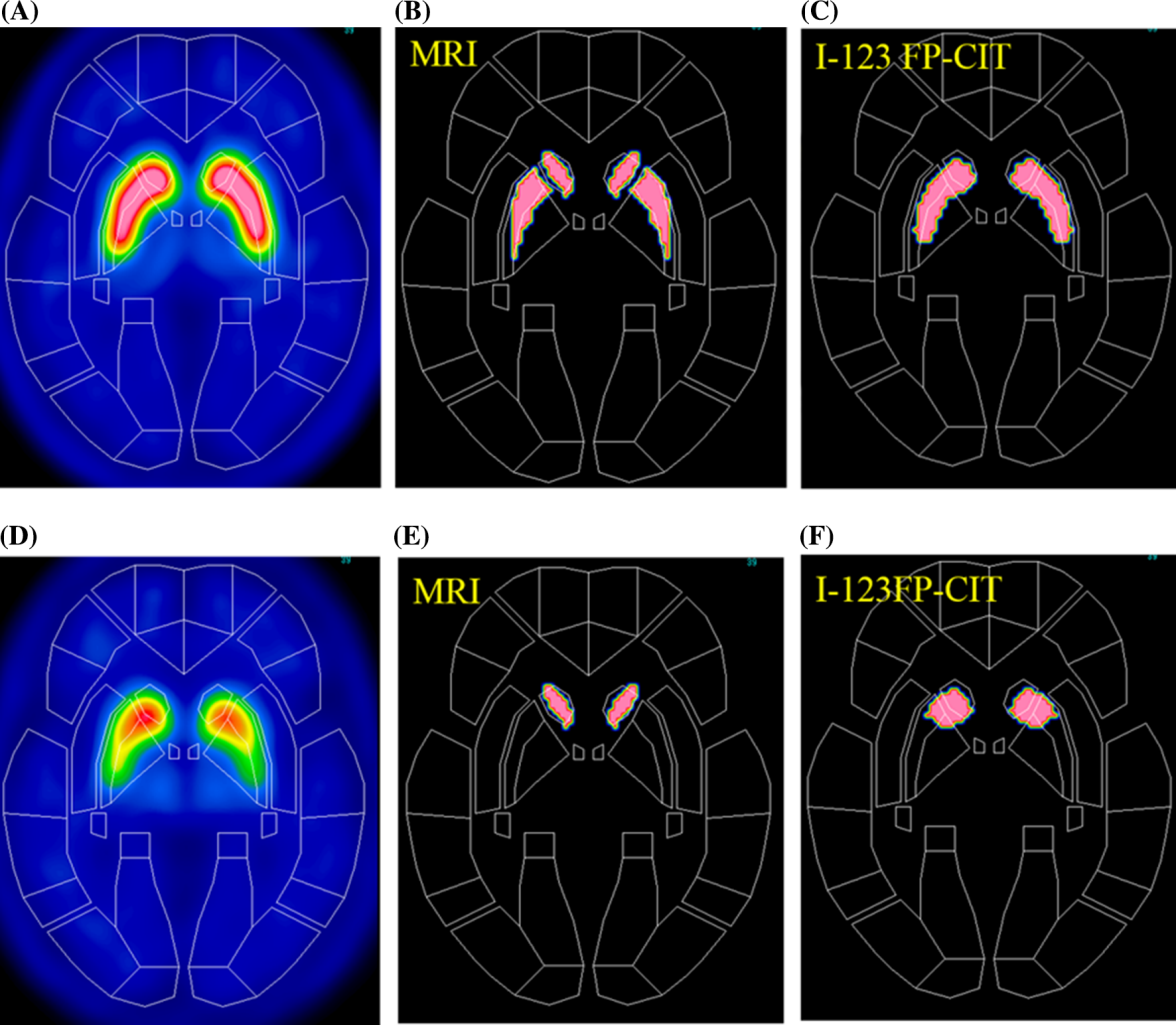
Magnified images of normal control subjects (a) and Parkinsonian patients (d) displayed using the FineSRT system: b, e MRI-based ROI, c, f I-123 FP-CIT-based ROI
